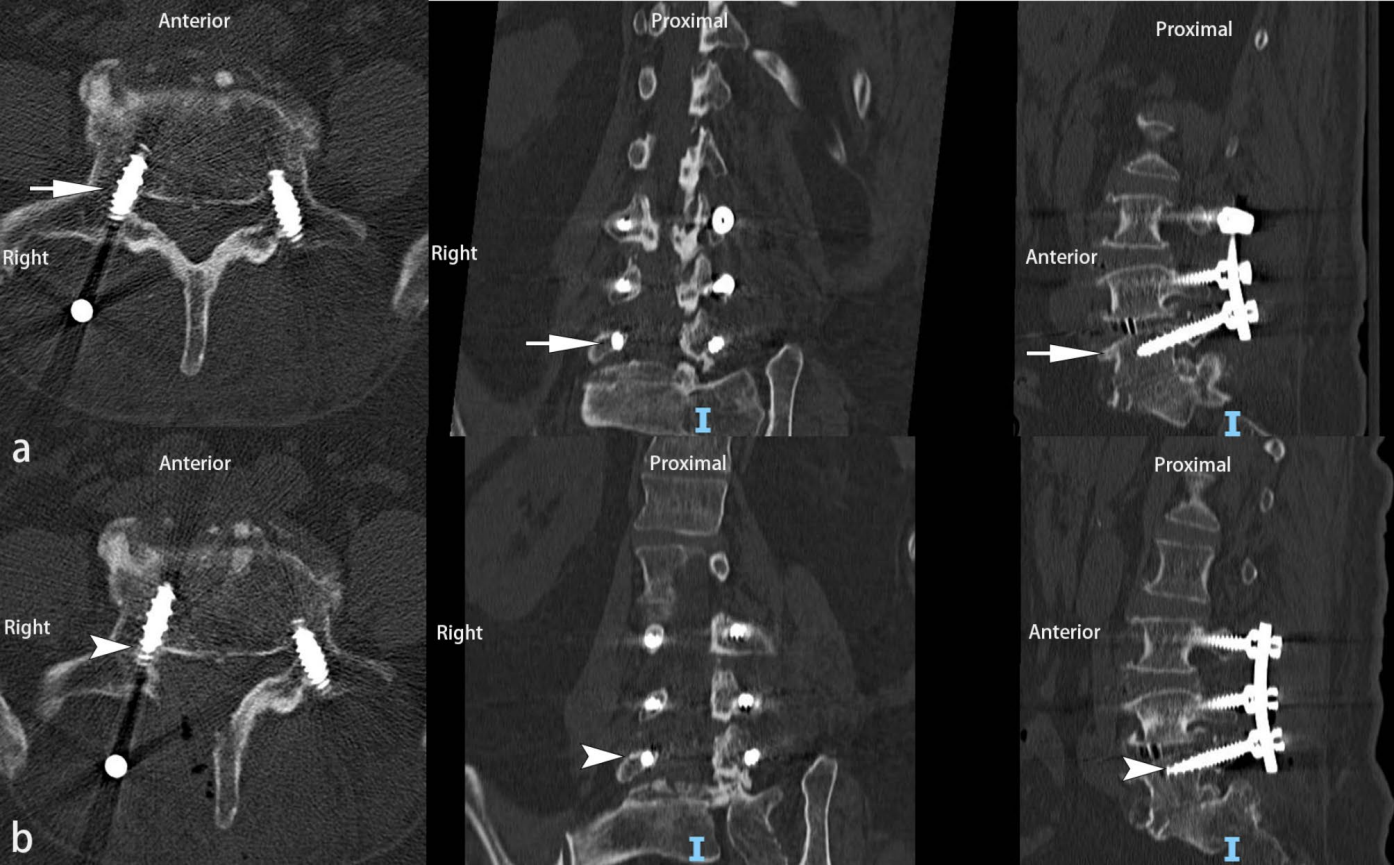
A 71-year-old woman underwent transforaminal lumbar interbody fusion (TLIF) from L3-L5. At postoperative day 1, the patient suffered persistent radiated pain from the back to the right foot. The postoperative CT scan showed that a minor medial screw breach (< 2 mm, grade 1) of the right L5 pedicle (a, white arrow). The patient received two weeks of conservative treatment without relief. The patient received a second surgery to revise the breached screw of L5 on the third postoperative week without T-EMG monitoring. Before removing the violated screw, the breached medial pedicle wall and the adjacent nerve root were clearly exposed, the nerve root was carefully retracted to central canal to avoid being curled by backing rotated screw. After re-inserting the screw, in addition to the use of the intraoperative X ray, the medial pedicle wall and the adjacent nerve root were confirmed again to avoid directly contact. The radiated pain was entirely resolved after the revision surgery. The CT scan showed a revised screw without the pedicle wall breach (b, white arrowhead)
